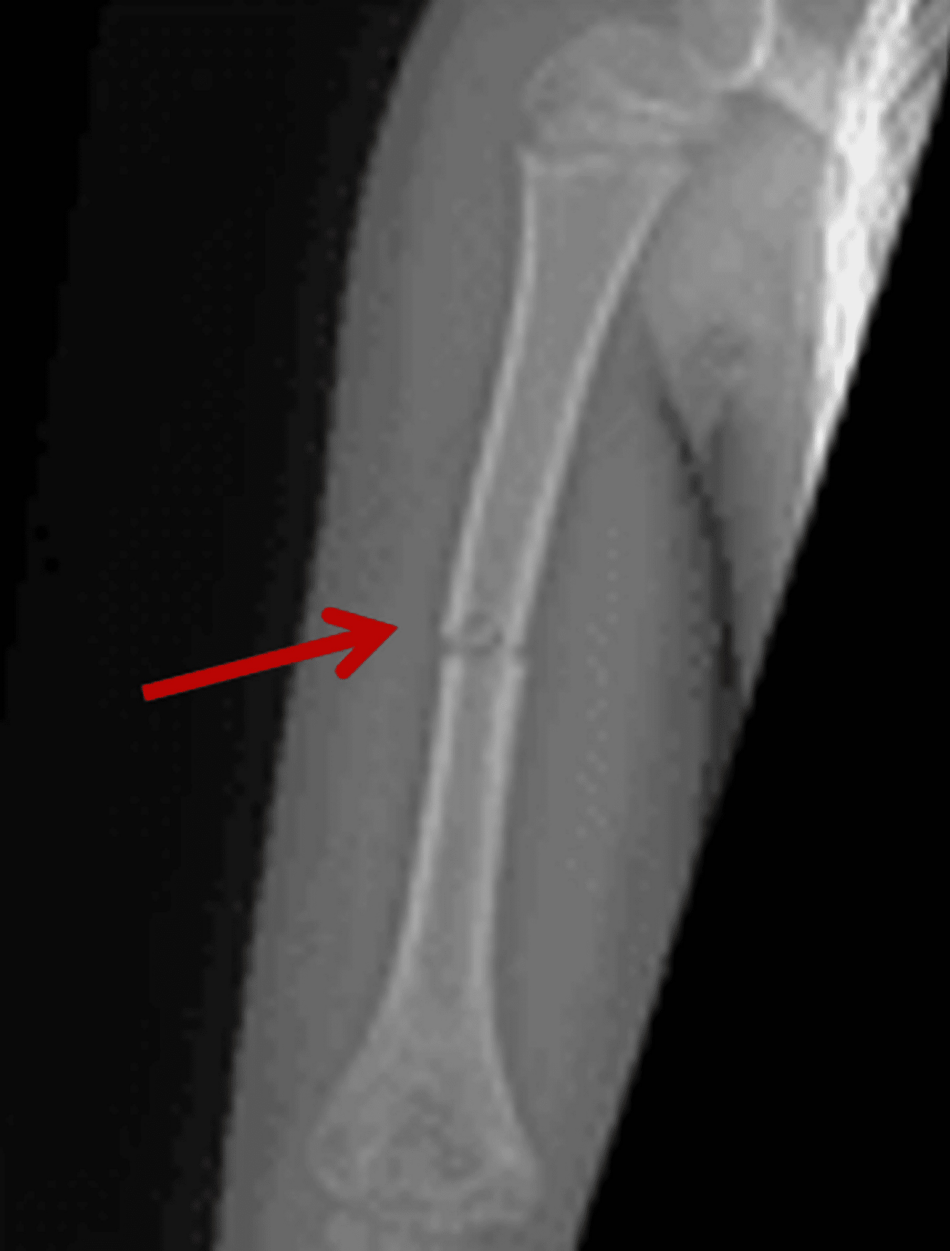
X-ray showing a fracture of the humerus (arrow)
